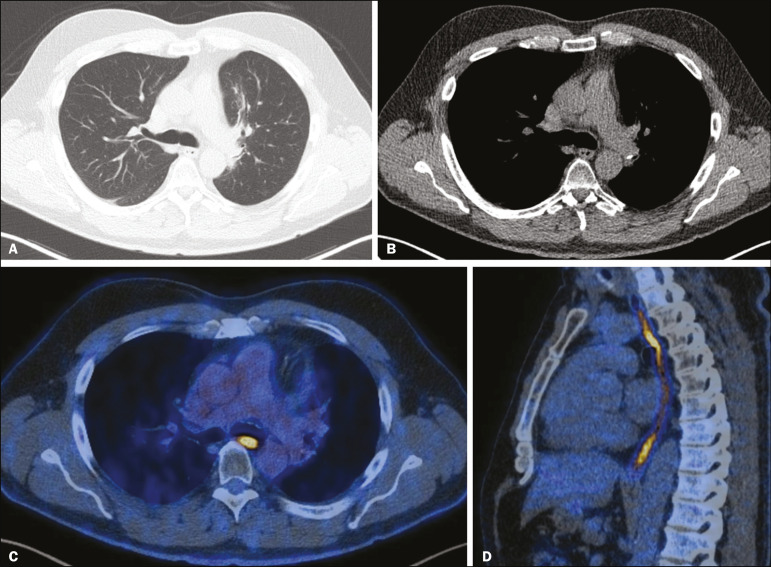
CT scans of a patient who had undergone radiotherapy for the treatment of lung cancer, showing retractile, ground-glass opacities in the left perihilar region (in A) and slight thickening of the esophageal walls (in B). Axial and sagittal 18F-FDG PET/CT slices (C and D, respectively), showing increased metabolic activity in the esophagus, suggestive of a radiation-induced inflammatory process (esophagitis).
